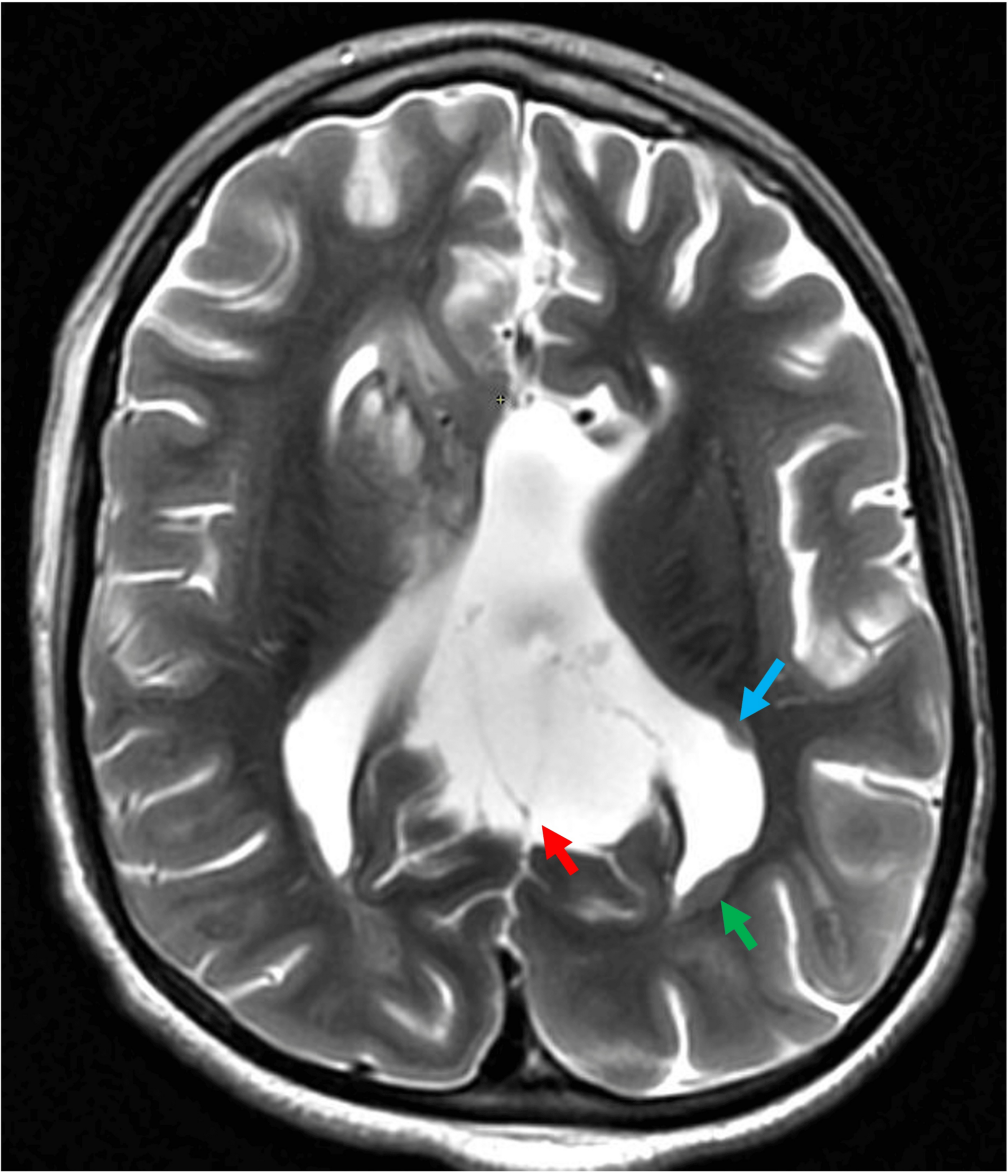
Corpus callosum agenesis with interhemispheric cyst. Axial T2-weighted imaging showing interhemispheric cyst (red arrow), associated with parallelly oriented ventricles (green arrow). Focal periventricular gray matter heterotopia (blue arrow) was noted.
